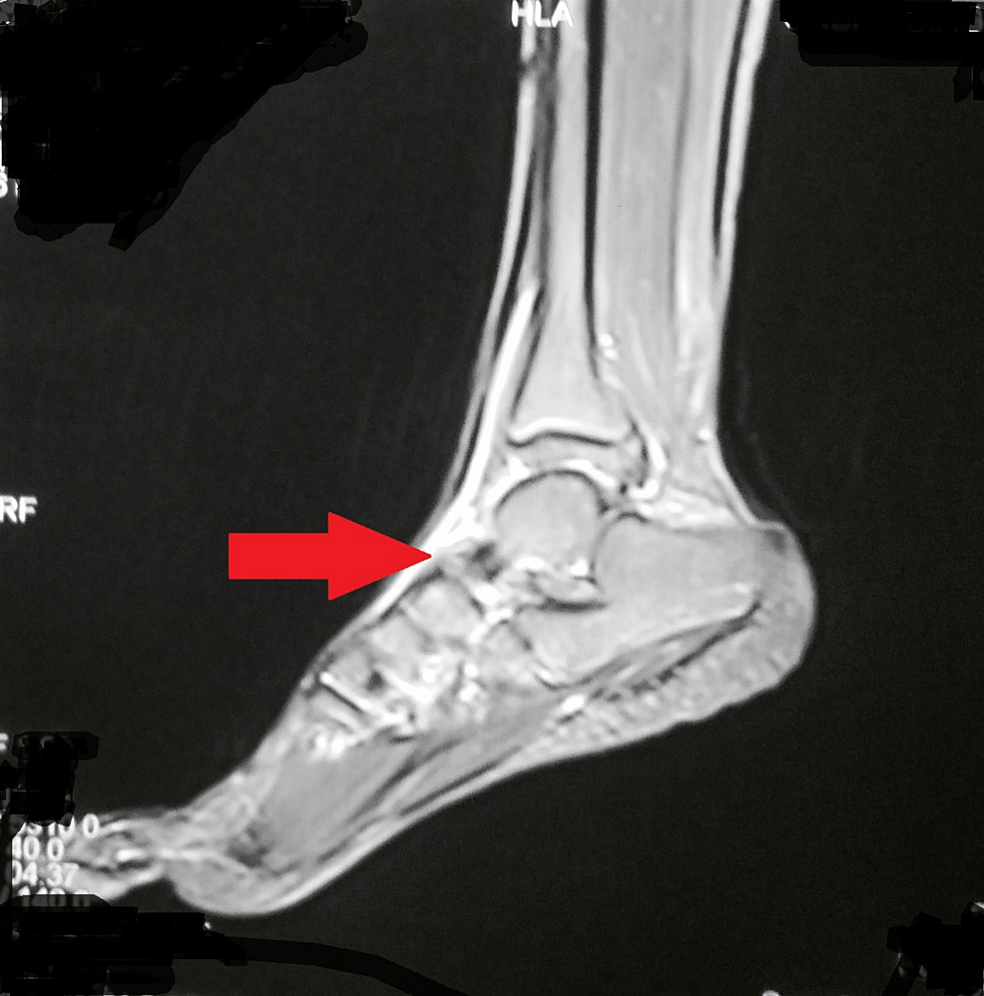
Magnetic resonance imaging of the left foot suggestive of focal erosion of the medial navicular bone
